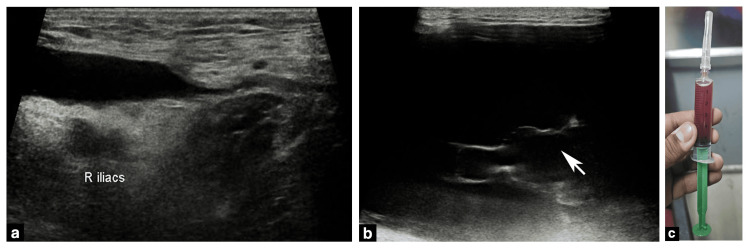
(a, b) Emergent ultrasound (US) examination revealed a large anechoic fluid collection in the subcutaneous plane of the right hip with (b) incomplete internal septations (white arrows). (c) US-guided aspiration yielded a serosanguinous fluid. (a) Right iliac vessels outline the medial border of the collection. (b) White arrow points to incomplete internal septations within the collection
